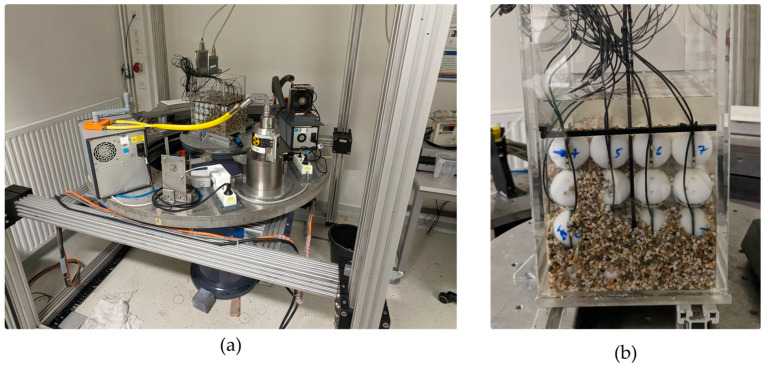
Photographs of the GravelSens calibration experiment: (a) the entire setup, including the CT scanner; (b) a detailed view of GravelSens partially filled with 3–5 mm sediments.
